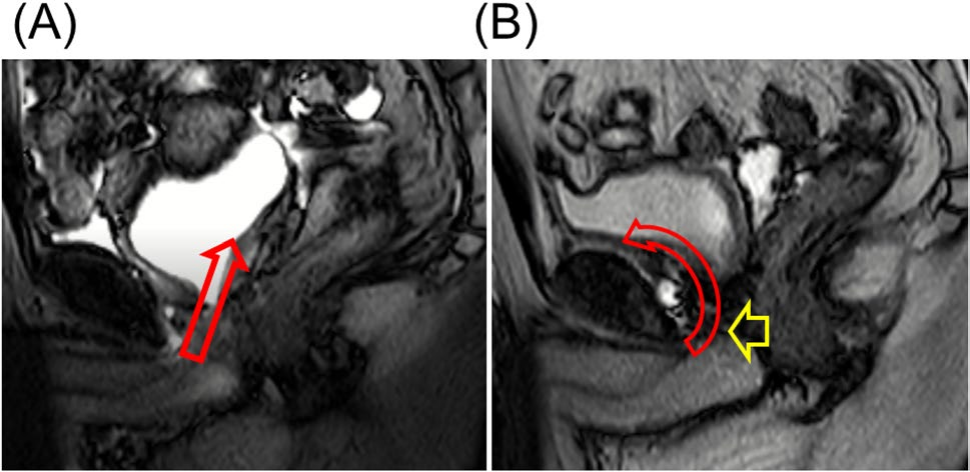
(A) Magnetic resonance imaging (MRI) after conventional robot-assisted radical prostatectomy (C-RARP). In C-RARP, the urethrovesical anastomosis is thought to be pulled cephalodorsally (red arrow) because the bladder vasculature is fixed from both dorsolateral sides. (B) MRI after Retzius-sparing RARP (RS-RARP). In RS-RARP, the anterior bladder wall is widely fixed, and the urethrovesical anastomosis is thought to be pulled cephalad ventrally (red arrow). After RS-RARP, the urethra is pushed in the direction of the pubic bone (yellow arrow), and the urethral closure pressure at rest may be higher than that after conventional RARP.
